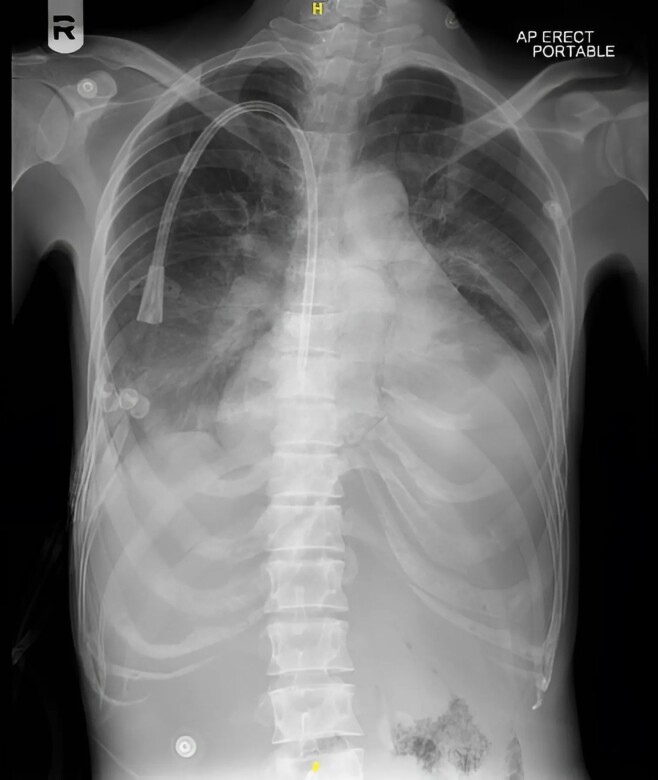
Chest X-ray revealed cardiomegaly, increased pulmonary vascular markings, mild pleural effusion, and a dialysis catheter *in situ*.
